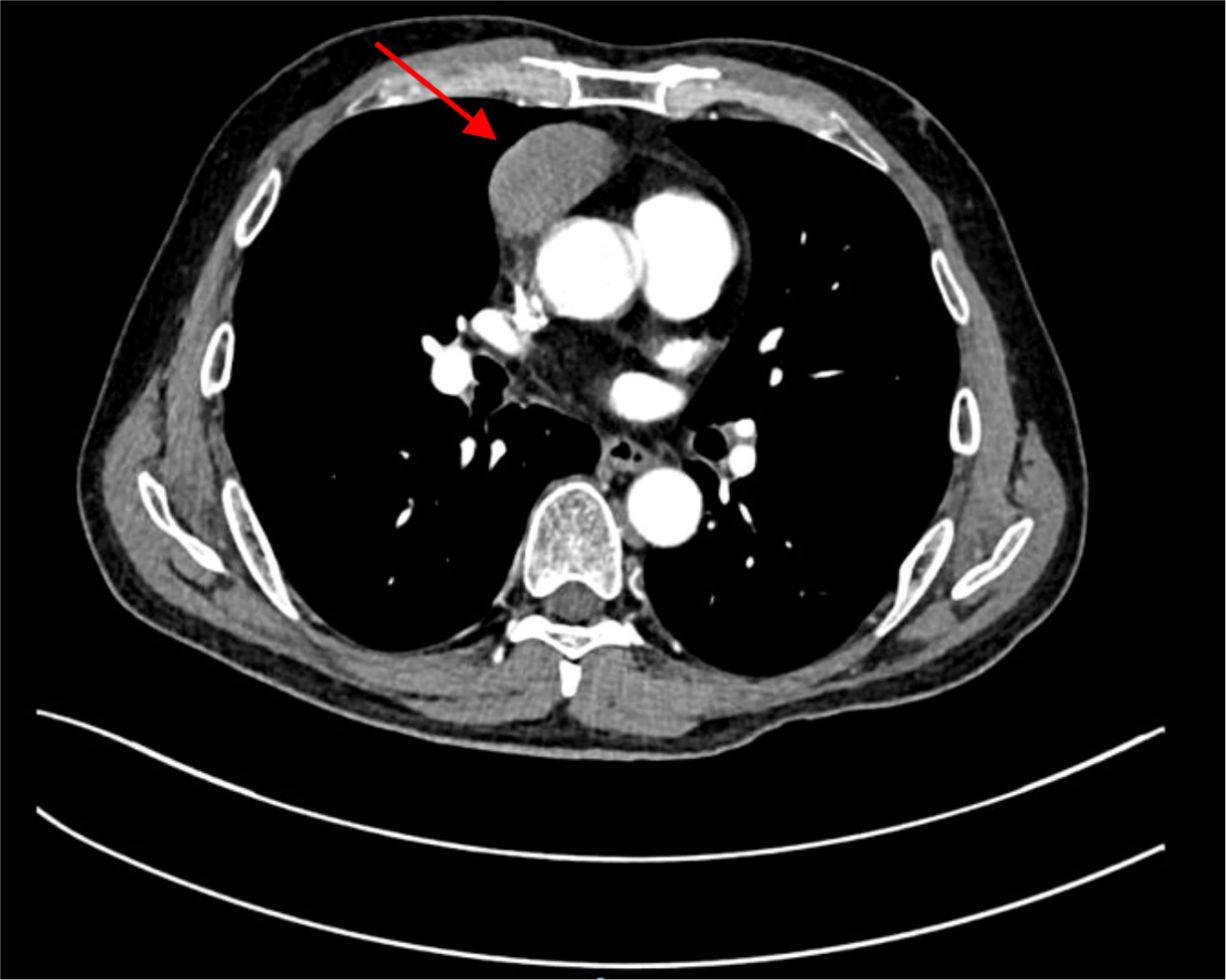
Contrast-enhanced chest CT scan showing an anterior mediastinal mass. A 5.0 × 4.3 × 2.5-cm well-circumscribed, heterogeneously enhancing mass is present in the anterior mediastinum (arrow), consistent with thymoma. The lesion abuts but does not invade adjacent great vessels.
